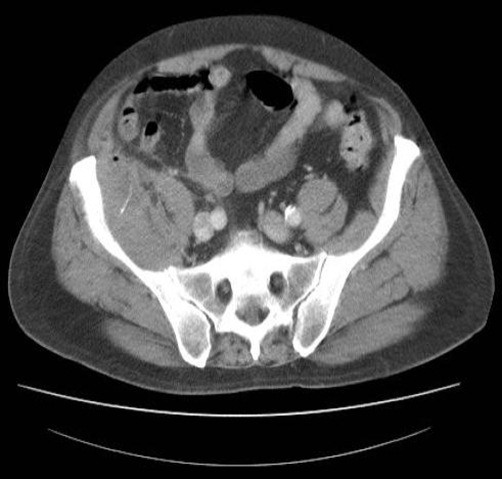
CT scan of the abdomen showing an appendix mass with a small abscess and a linear opaque foreign body.
